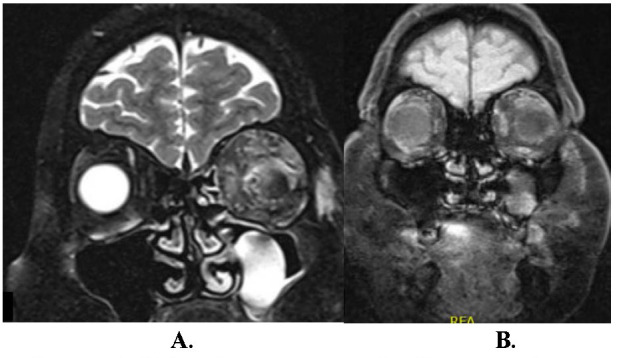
MRI, coronal scans. A. Before immunosuppressive therapy - enlargement of left lateral, superior and medial extraocular muscle. B. Decrease in thickness after immunosuppressive therapy
